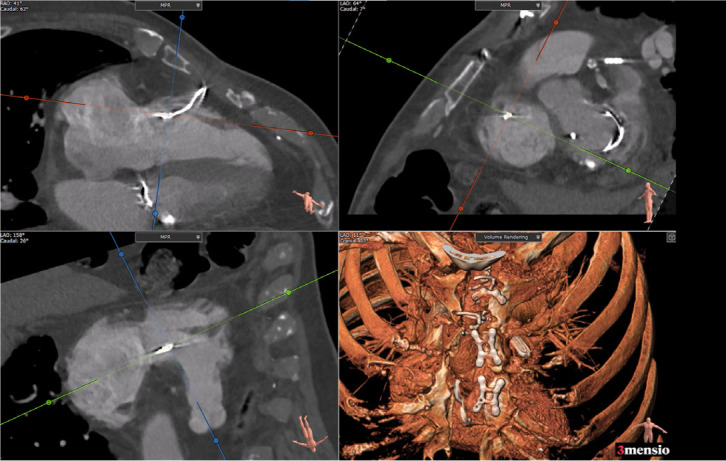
Multiplane chest computed tomography angiogram: fragmented sternal
